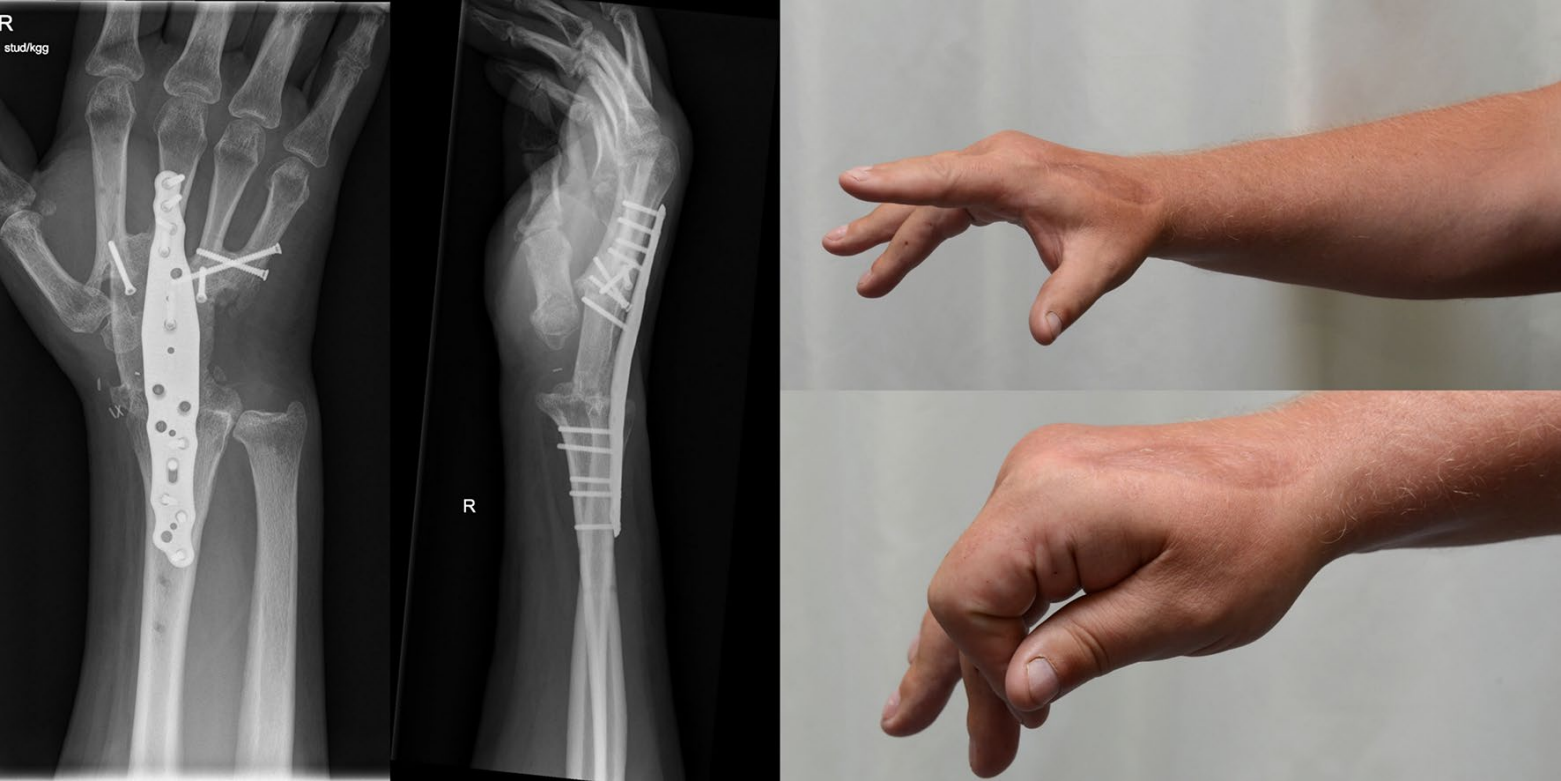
a 12 months follow-up radiographs showing bony union of the arthrodesis. Acceptable and stable alignment of the first digital ray without any further proximalization, resulting in a limited but satisfactory range of motion for the patient with the ability to perform a pinch grip (b)
